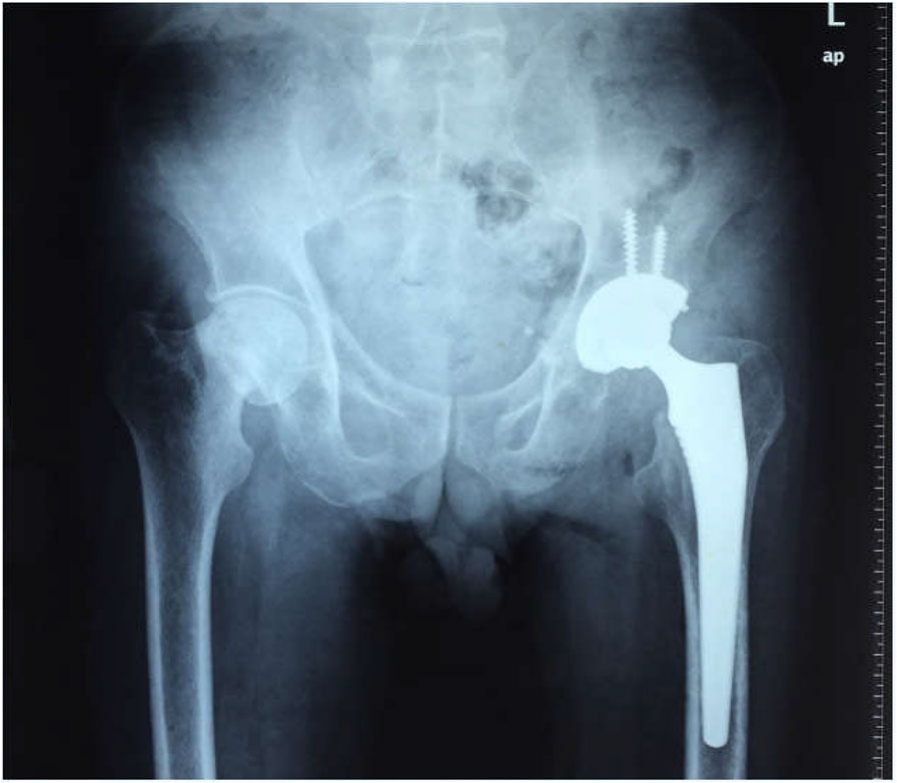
Postoperative radiograph of the pelvis demonstrating the success of the left total hip replacement
